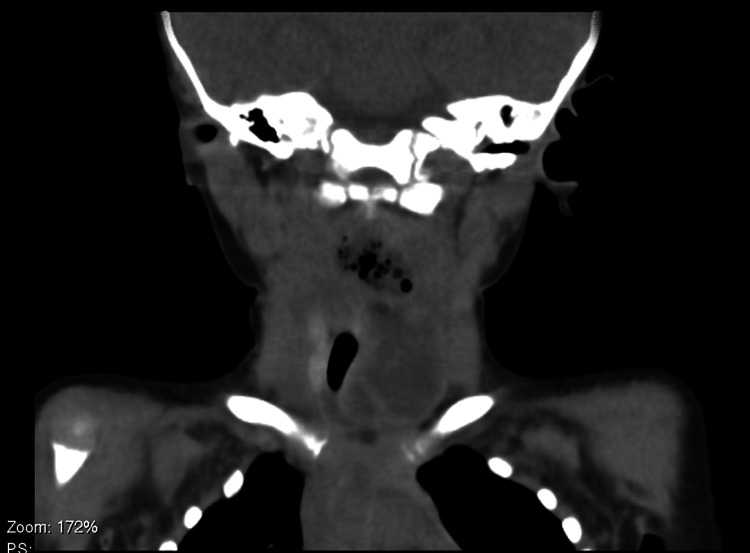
Cervical CT scan: coronal view A cervical CT scan revealed a thyroid abscess, measuring 4.3x1.5x2.4 cm, with interior gas bubbles suggestive of anaerobic infection. CT: computed tomography
